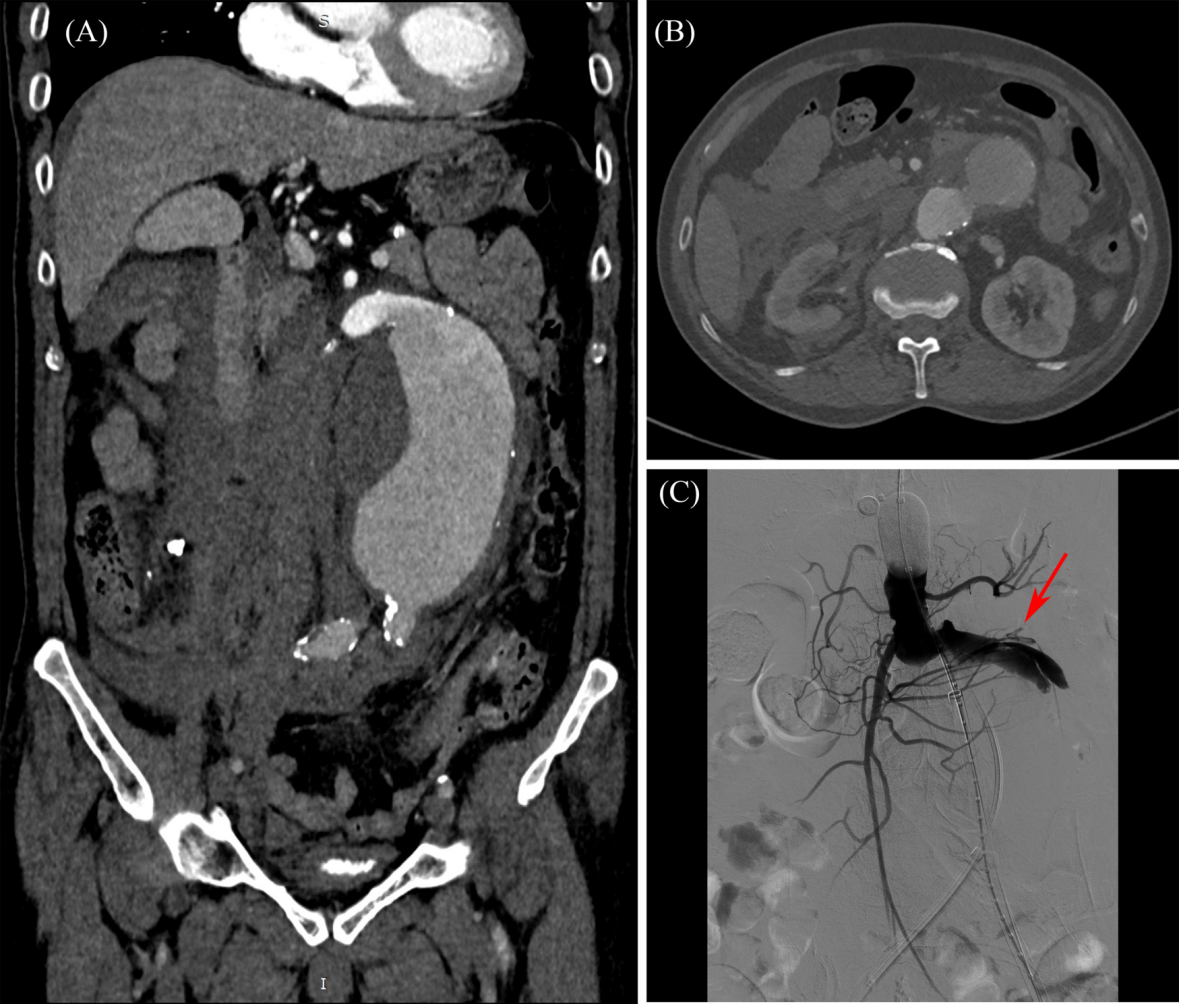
Preoperative and intraoperative imaging of the abdominal aortic aneurysm. (A) Preoperative CTA–coronal view; (B) preoperative CTA–cross-sectional view at aneurysm neck level; (C) intraoperative DSA–Rupture site captured by DSA imaging.
